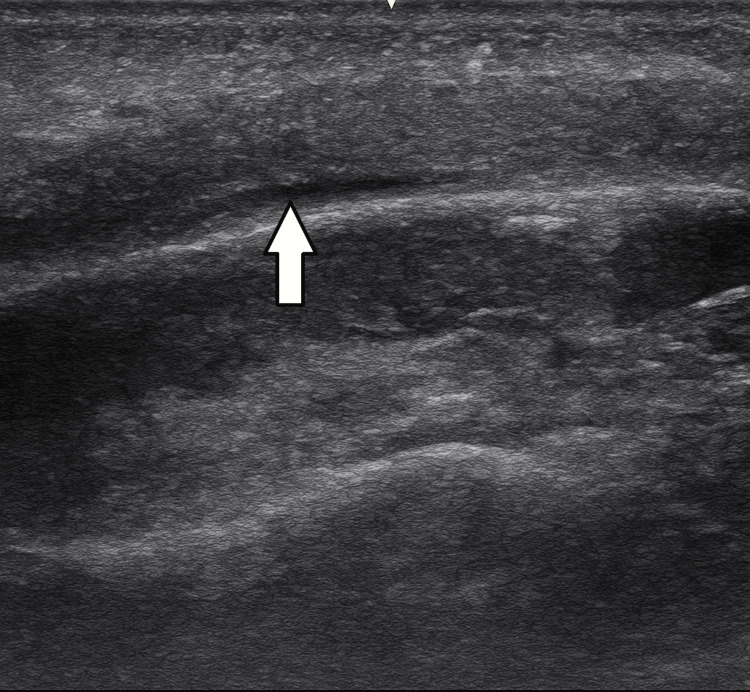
Point-of-care ultrasound showing a thin subperiosteal fluid collection (white arrow).
